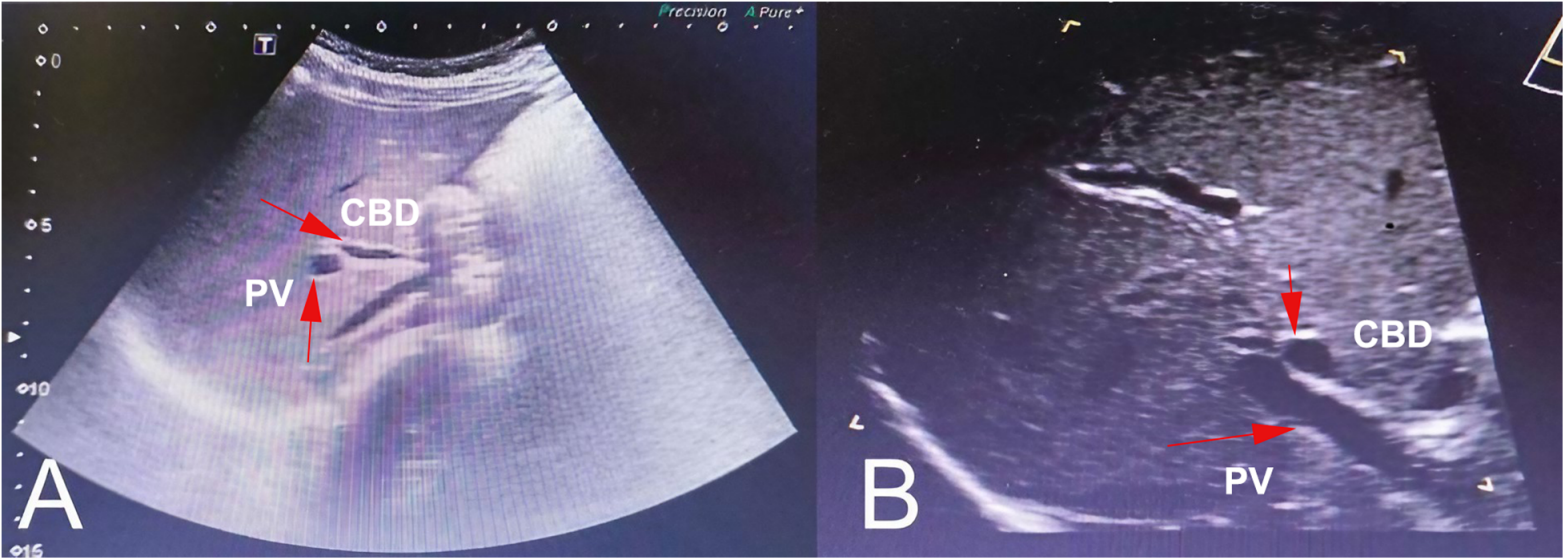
Ultrasound scans of a pediatric patient with CAGB. An ultrasonographic image (A) and after magnification (B) of the gallbladder showed a deficiency in the gallbladder. The common bile duct (CBD) and portal vein (PV) are indicated by arrows for reference. No gallbladder or gallbladder remnant was visible in the expected location.
